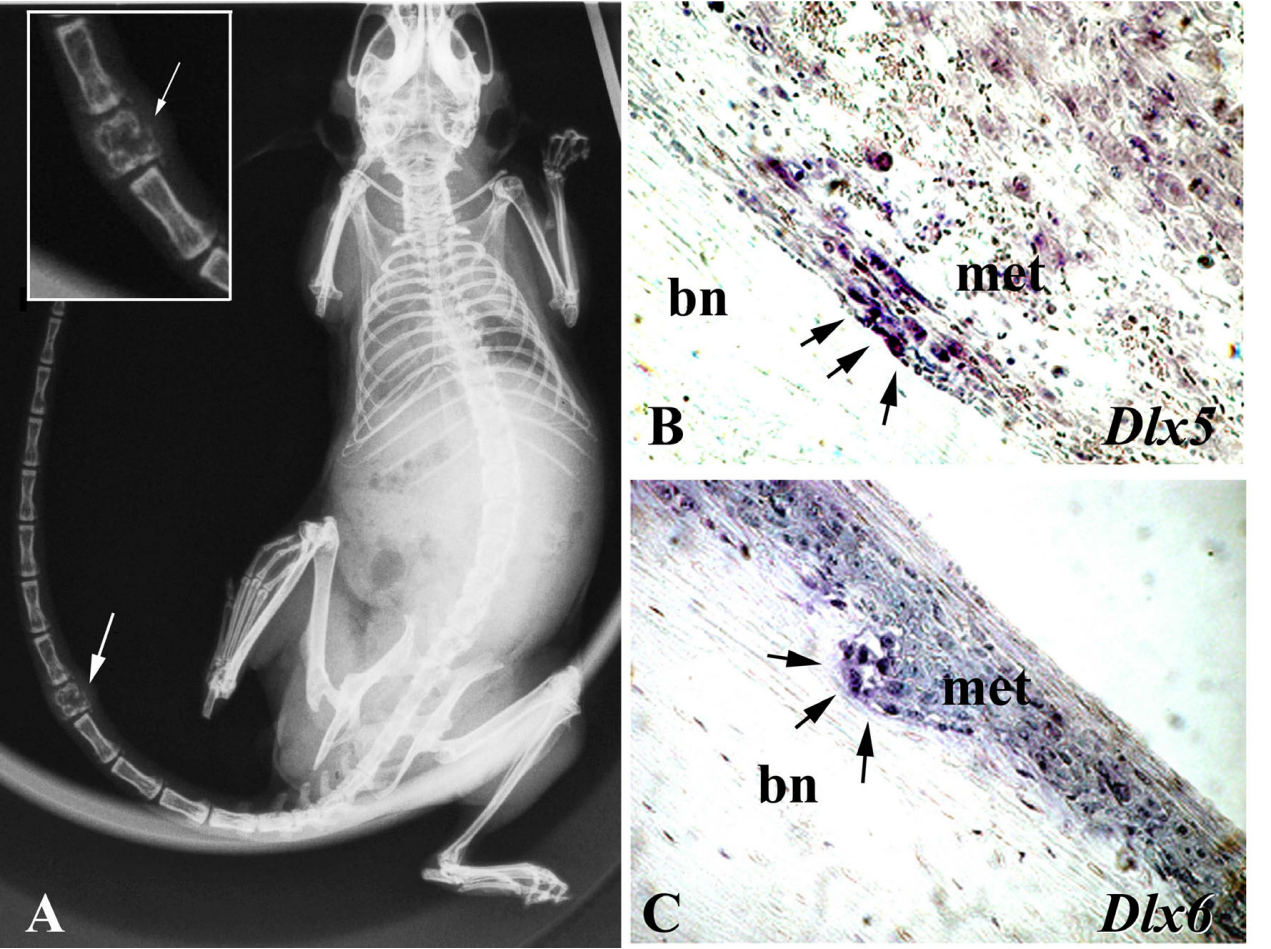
In situ *hybridization for *DLX5 *and *DLX6 *in MDA-MB-231 bone metastases. (A) A bone metastasis, visualized by radiography. (B and C) In-situ hybridization on sections of long bones containing MDA-MB-231 metastases showing high expression of *DLX5 *and *DLX6*. The signal was more intense at the invasion front (arrows). bn, cortical bone; met, metastasis.
